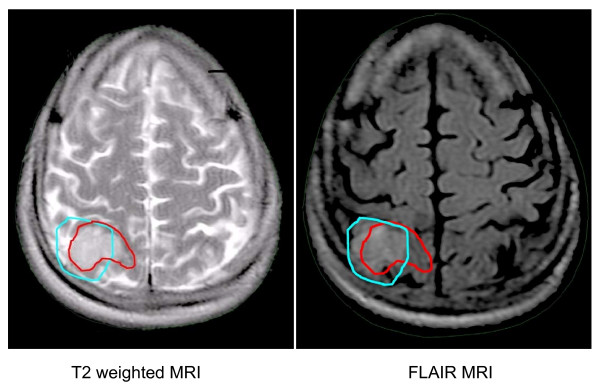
Overlay of tumor volumes as contoured on T2 and FLAIR sequences. The T2 abnormality is contoured in red and the FLAIR abnormality is contoured in cyan.
